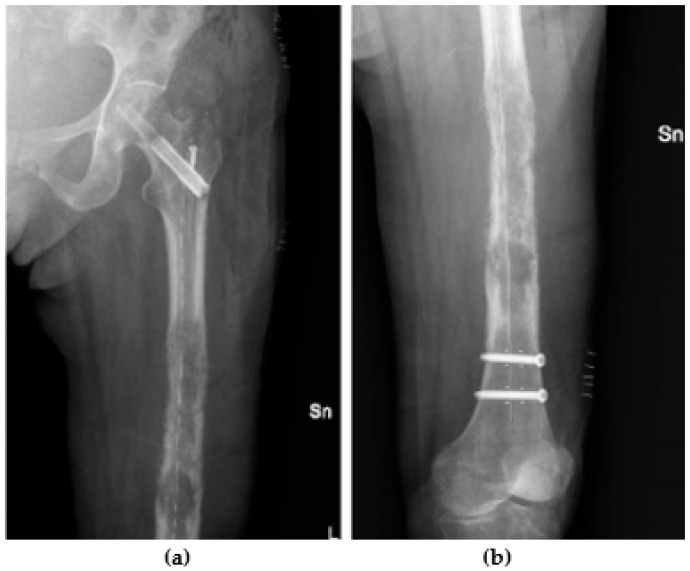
X-ray of a metastatic lesion from pancreatic adenocarcinoma in the left femur in a 74-year-old woman treated with a CFR nail: (a) X-ray view of proximal femur; (b) X-ray view of distal femur.
